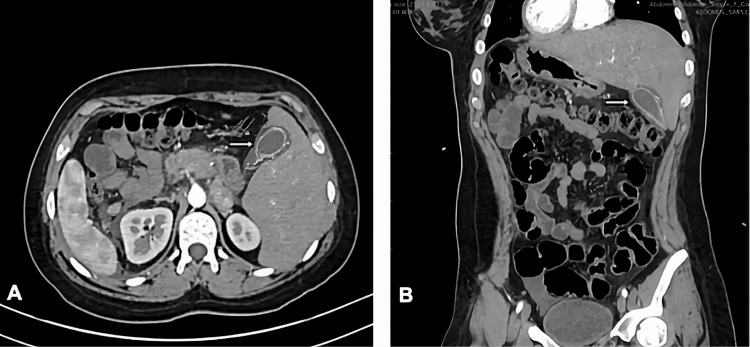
Contrast-enhanced computed tomography confirming situs inversus totalis. Contrast-enhanced computed tomography confirming situs inversus totalis. (A) Axial image demonstrating complete mirror-image transposition of the abdominal viscera. (B) Coronal reconstruction showing dextrocardia and a left-sided liver and gallbladder with inflammatory changes consistent with acute calculous cholecystitis (white arrows).
